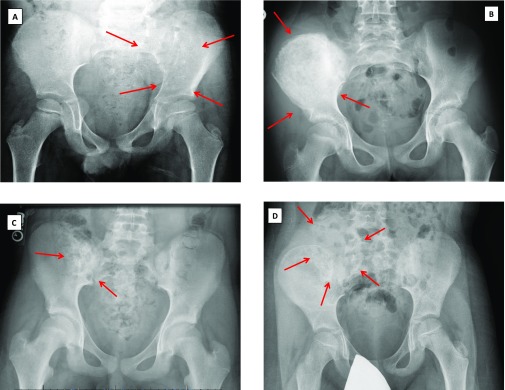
Radiographic presentation of pelvic sarcoma. Lytic lesion in 6.7-year-old male with Ewing sarcoma in the left ilium (A), calcific lesion in a 12 years old female with Ewing sarcoma in the right ilium (B), mixed lesion in a 14 years old male with Ewing sarcoma in the right ilium (C), and soft tissue mass without boney lesion in a 7 years old male with Ewing sarcoma (D).
